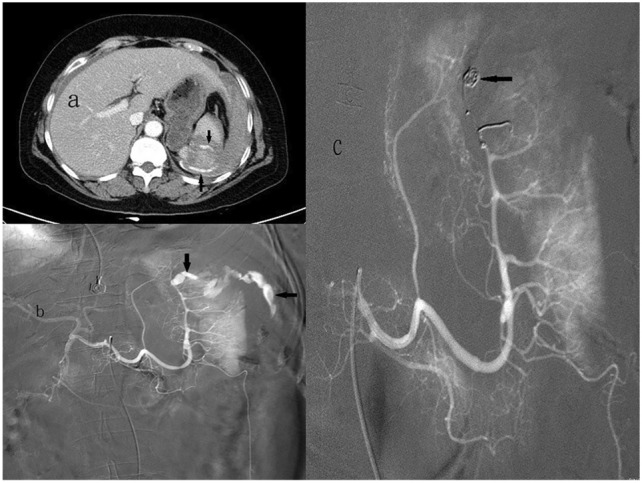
61-Year-old woman with a grade V blunt splenic injury underwent distal splenic arterial embolization. a Admission contrast-enhanced helical computed tomography scan demonstrates splenic laceration with perisplenic contrast medium extravasation (arrows). b The splenic angiogram demonstrates an active leak of contrast medium into the parenchyma with peritoneal cavity extension (arrows) from a branch artery of upper pole. c Metallic coils (arrow) were deployed selectively at upper pole. The post-embolization angiogram demonstrates no further extravasation from the injured spleen
